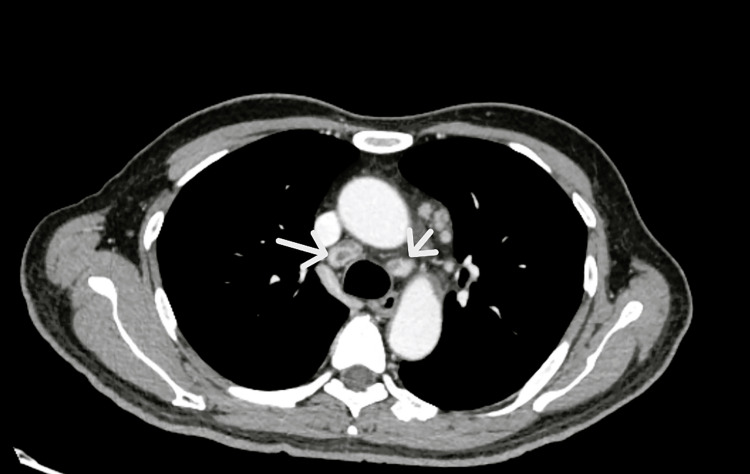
Chest computed tomography Chest computed tomography of the chest revealed necrotic-appearing, enlarged mediastinal lymph nodes (white arrows) with otherwise normal lung parenchyma.
